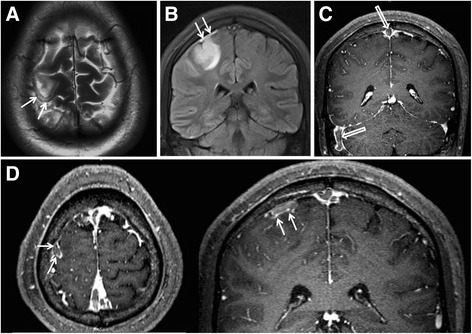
Typical images of the concomitant cortical vein thrombosis on conventional magnetic resonance imaging and contrast enhanced three dimensional-magnetization prepared rapid acquisition with gradient echo (CE-3D-MPRAGE) in a 23-year-old male patient with cerebral venous sinus thrombosis. The images of T2-weighted imaging (T2WI, a) and fluid-attenuated inversion recovery (FLAIR, b) show a cord-like hyperintensity (white arrows, representing a sub-acute thrombosis) of a right cortical vein. Venous infarction can be observed on FLAIR (b). The thin-thickness images of multiplanner reconstruction based on CE-3D-MPRAGE source images reveal the filling defects of contrast agent in the superior sagittal sinus and the right sigmoid sinus (c, hollow white arrows) and in the site of the aforementioned cortical vein (d, white arrows) presenting with a cord-like hyperintensity on T2WI and FLAIR
